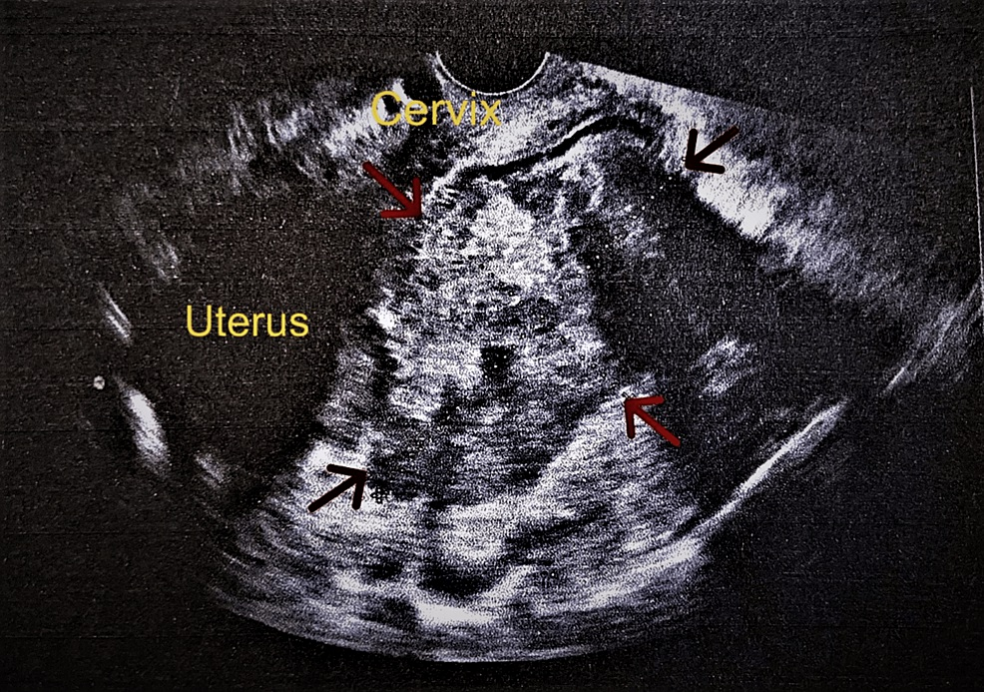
Transvaginal ultrasound imaging of a twisted mature cystic ovarian teratoma In the posterior pouch of Douglas, a solid inhomogeneous lesion is evident (red arrows), without cystic components and without the diagnostic imaging feature of intratumoral fat components forms that were misdiagnosed as a pelvic inflammatory disease.
